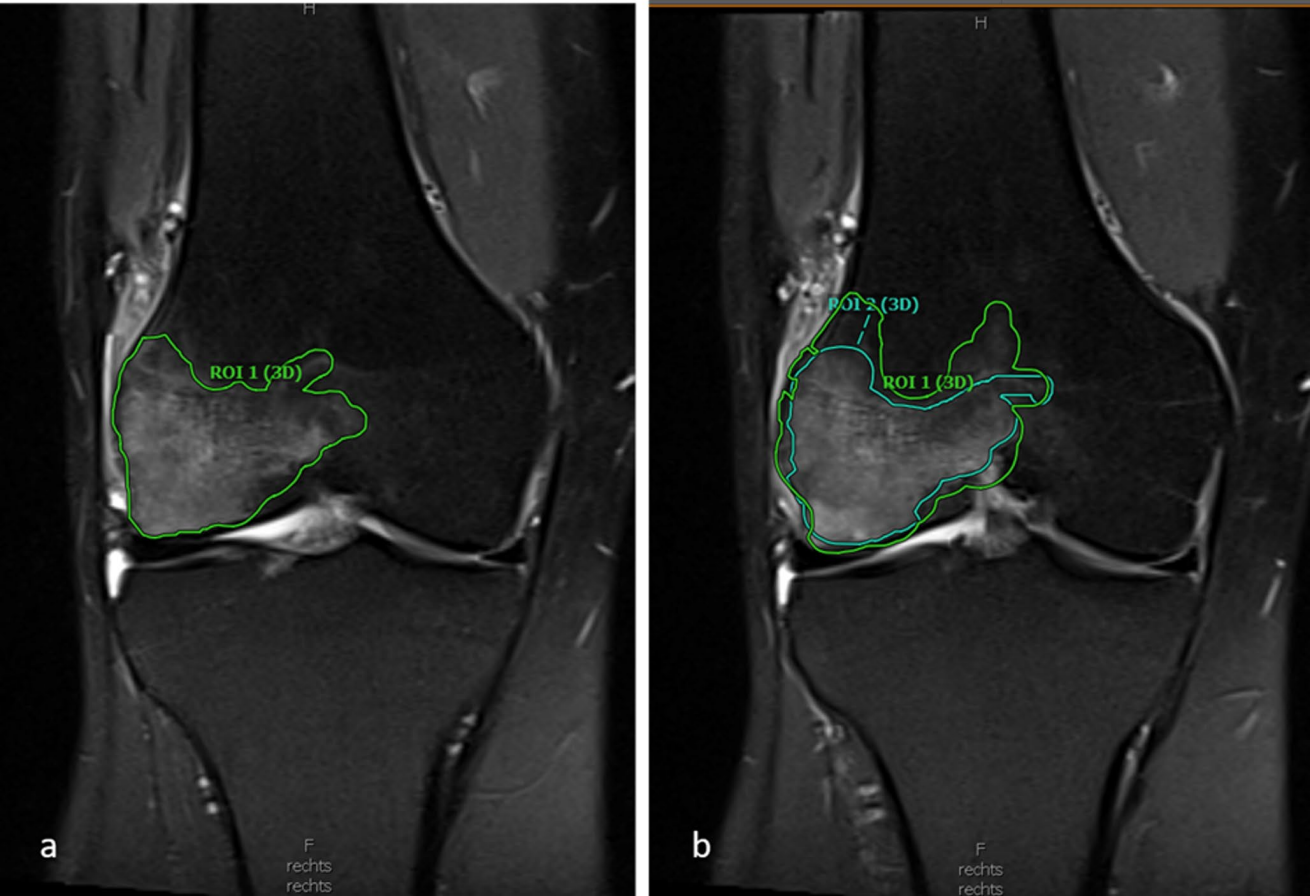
(a) of region of interest (ROI) of reader 1 in pre-treatment MRI (PD fs, coronal slicing, 3 mm thickness); (b) comparison of ROI delineation between observer 1 and 2, which used different threshold signal intensities to determine delineation
